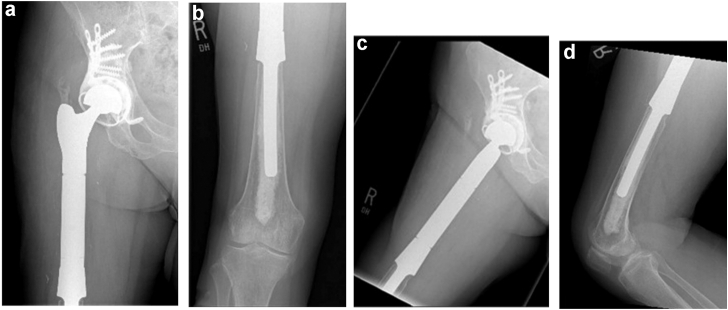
(a) Right hip anterior posterior (AP), (b) right distal femur AP, (c) right hip frog leg lateral, and (d) right distal femur lateral radiographs 5-year status postproximal femur replacement and acetabular reconstruction.
